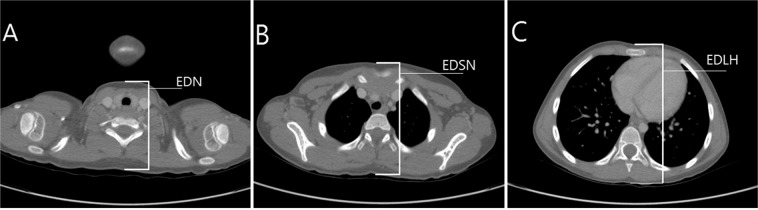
Axial image of the neck (A), sternal notch level (B), and at the lower half level of the sternum (C) from a computed tomography scan and the method for measuring each external anteroposterior diameter. EDN: external anteroposterior diameter of the neck. EDSN: external anteroposterior diameter of the chest at the sternal notch. EDLH: external anteroposterior diameter of the chest at the lower half of the sternum.
